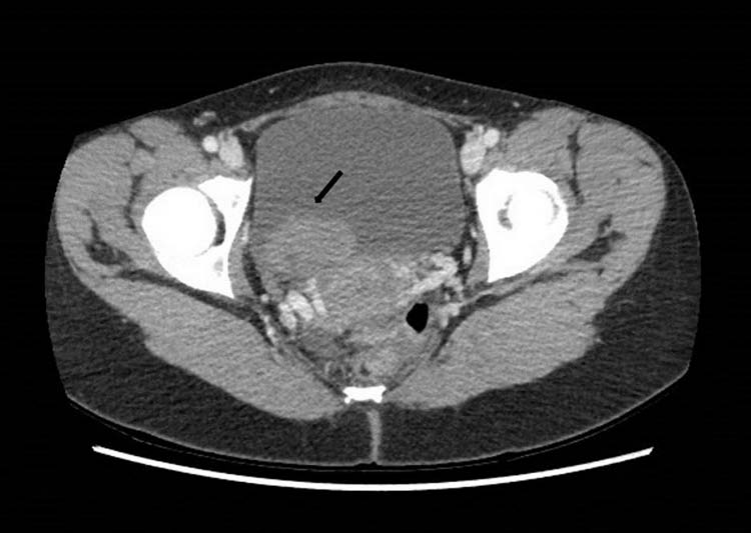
Computed tomography on presentation showing a bladder mass (arrow).
